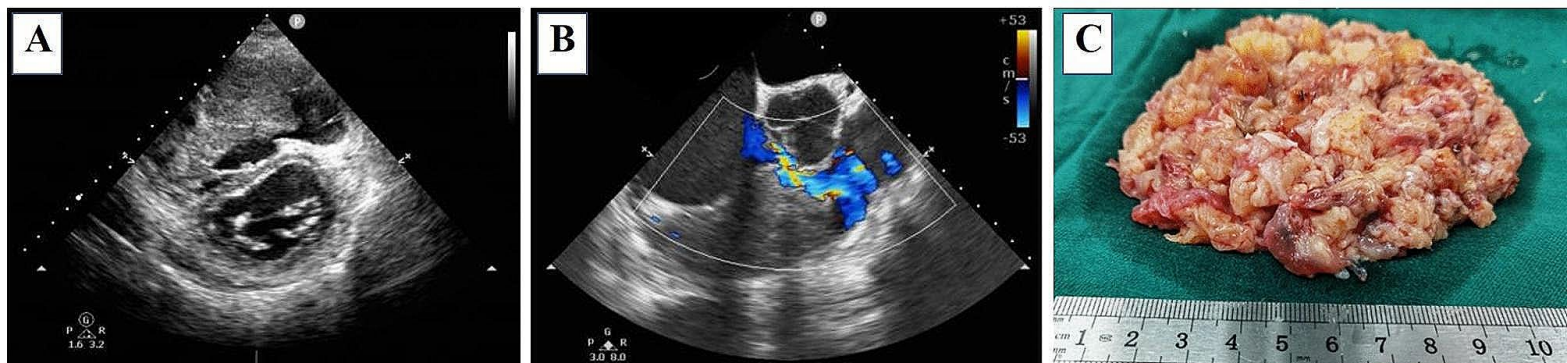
(A) and (B) represent transthoracic short axis section of LV and the mid-esophageal RV inflow-outflow view. Both TTE and TEE showed a huge mass occupying the inflow tract and most of the right ventricular cavity. The mass fused and wrapped with the tricuspid valve, and the demarcation between the tumor and the myocardium was not clear. The patient had a narrow gap to provide forward blood flow during diastole. (C) shows the excised fragmented tumor tissue
